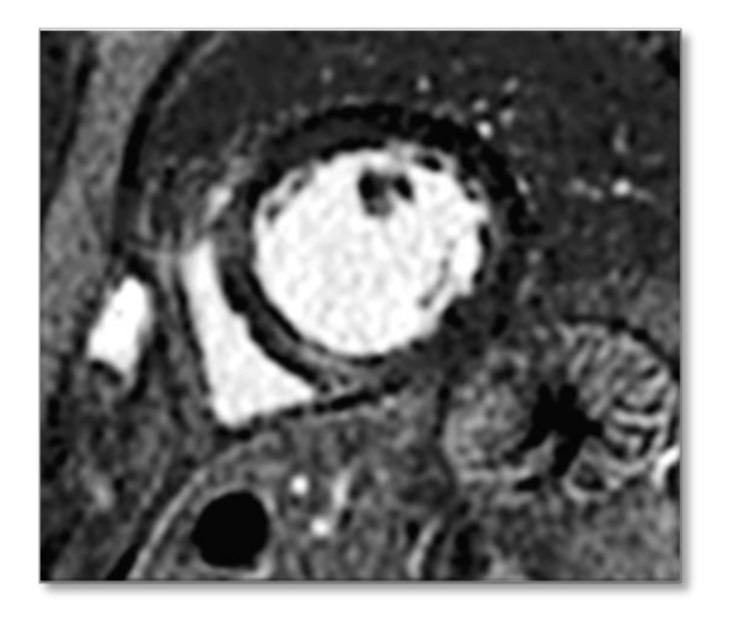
Magnetic resonance image (MRI) of the proband. The arrow shows intramyocardial late gadolinium enhancement (LGE) located in the inferoseptal wall.
